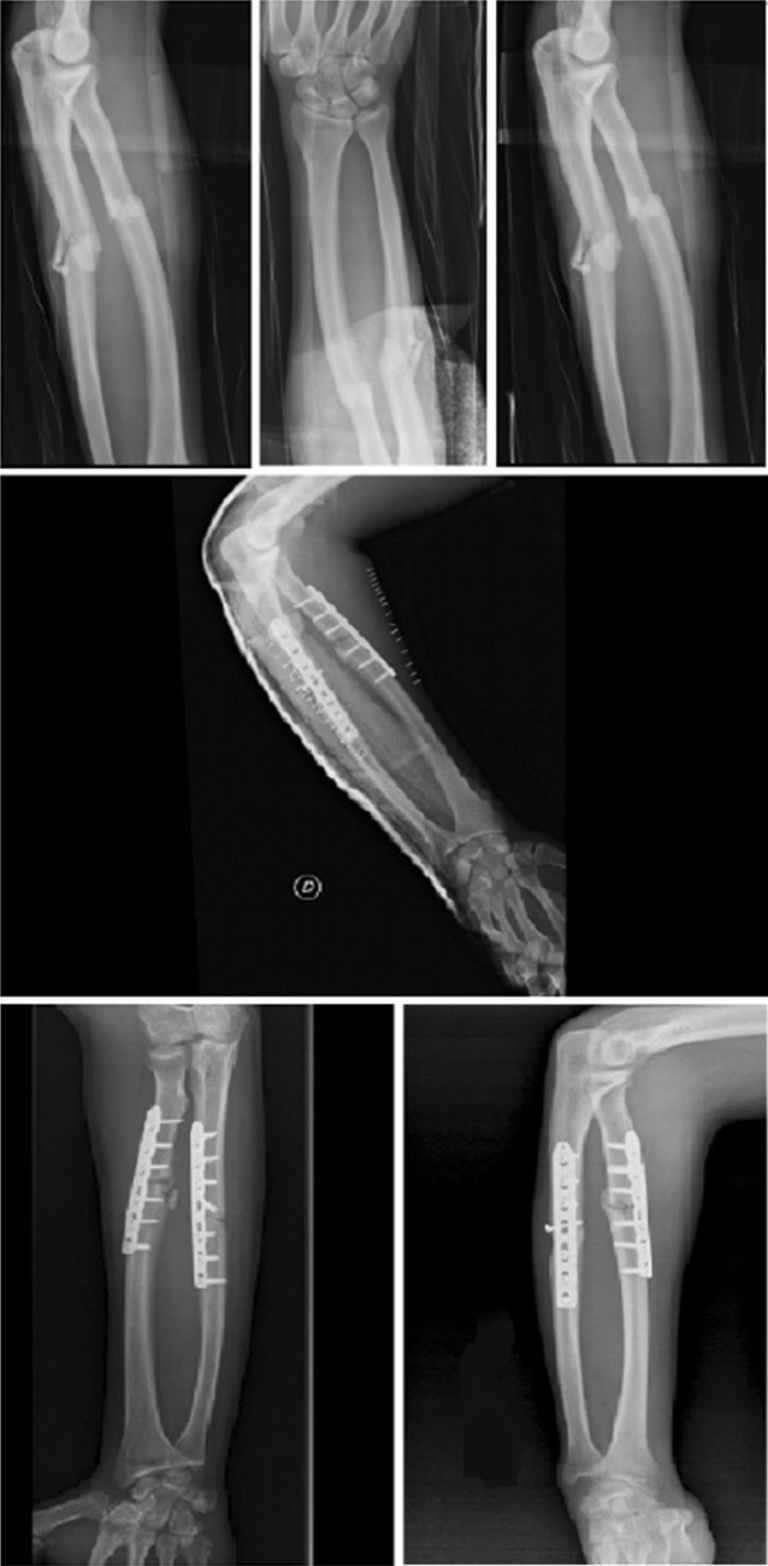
AP and lateral radiographic projections of post-operative treatment of a bi-osseous forearm fractures with two plates
